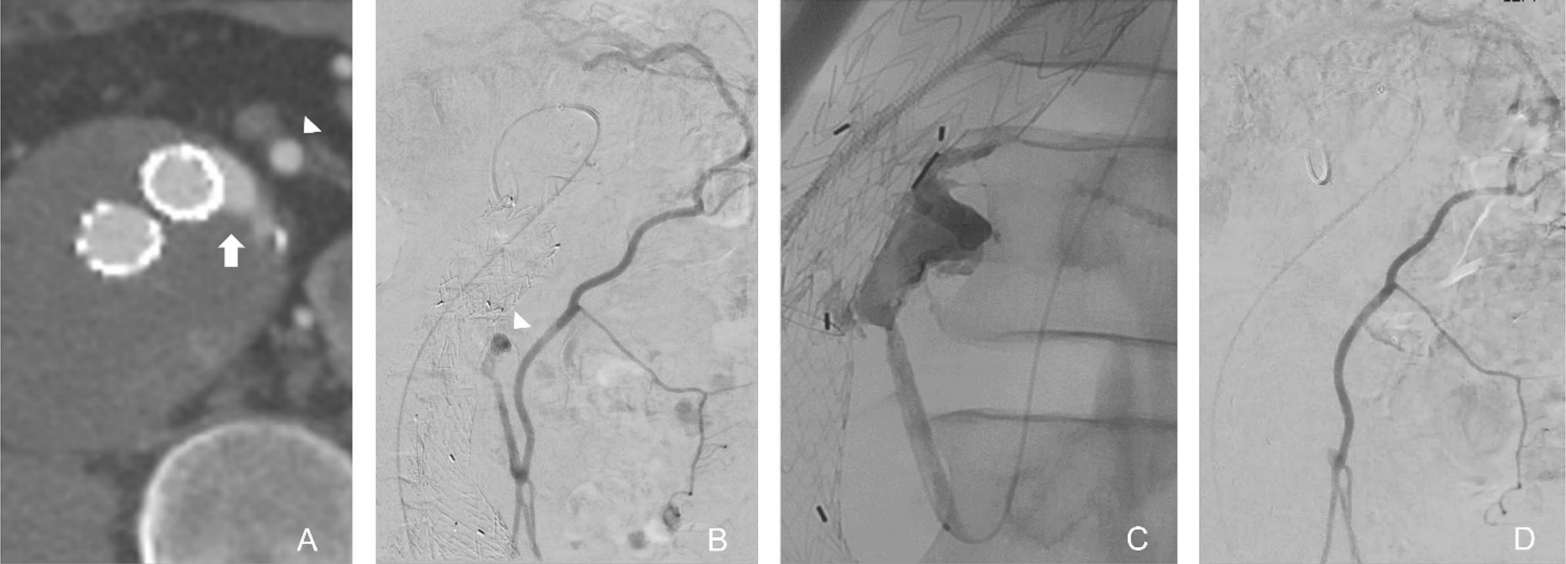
Transarterial embolisation of type II EL. (A) Axial CT image shows type II EL in the anterior sac (arrow) close to the IMA (arrowhead). (B) Angiogram from the middle colic branch of the SMA opacifies an hypertrophied arc of Riolan, IMA and EL cavity (arrowhead). (C) Embolisation with liquid embolic agent via a microcatheter. (D) Completion angiogram shows no further EL opacification
